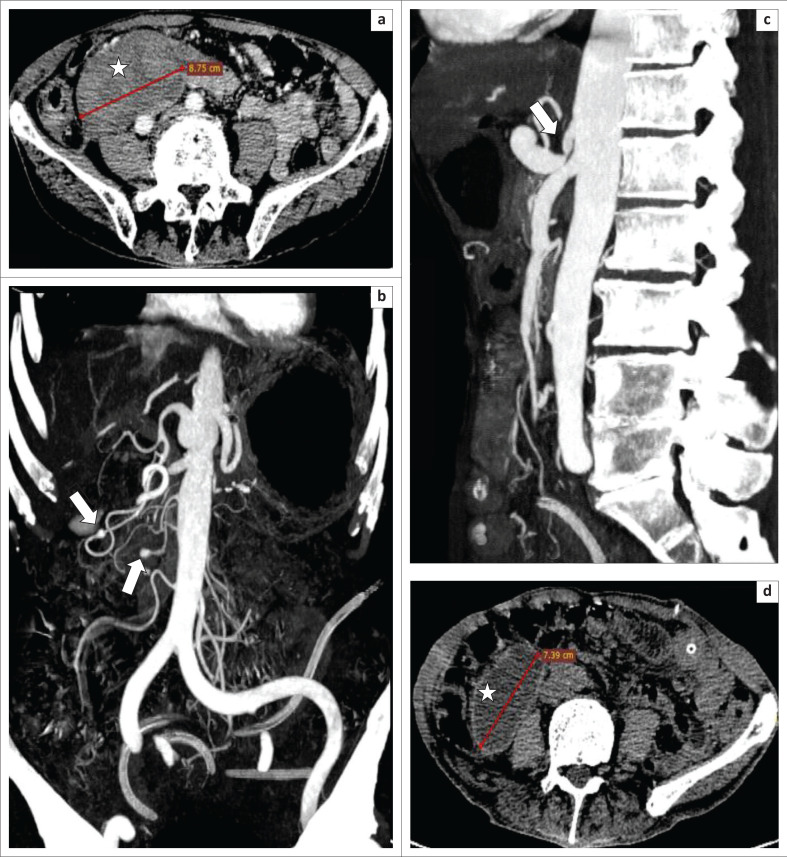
A 65-year-old man with atraumatic acute abdominal pain and vomiting: Arterial phase contrast enhanced computed tomography of the abdomen in the axial (a), coronal (b) and sagittal planes (c) show a well-orgnised mesenteric haematoma (asterix, a) of approximately 9 cm. Two small aneurysms were identified (arrows, b) originating from the upper and lower pancreatico-duodenal arteries. There was significant coeliac artery compression by the median arcuate ligament (arrow, c) resulting in post-stenotic fusiform dilatation of the celiac artery. Non-enhanced computed tomography in the axial plan (d), performed 2 months later, shows homogeous liquifecation of the haematoma with a well-distinguished regular and thin wall.
